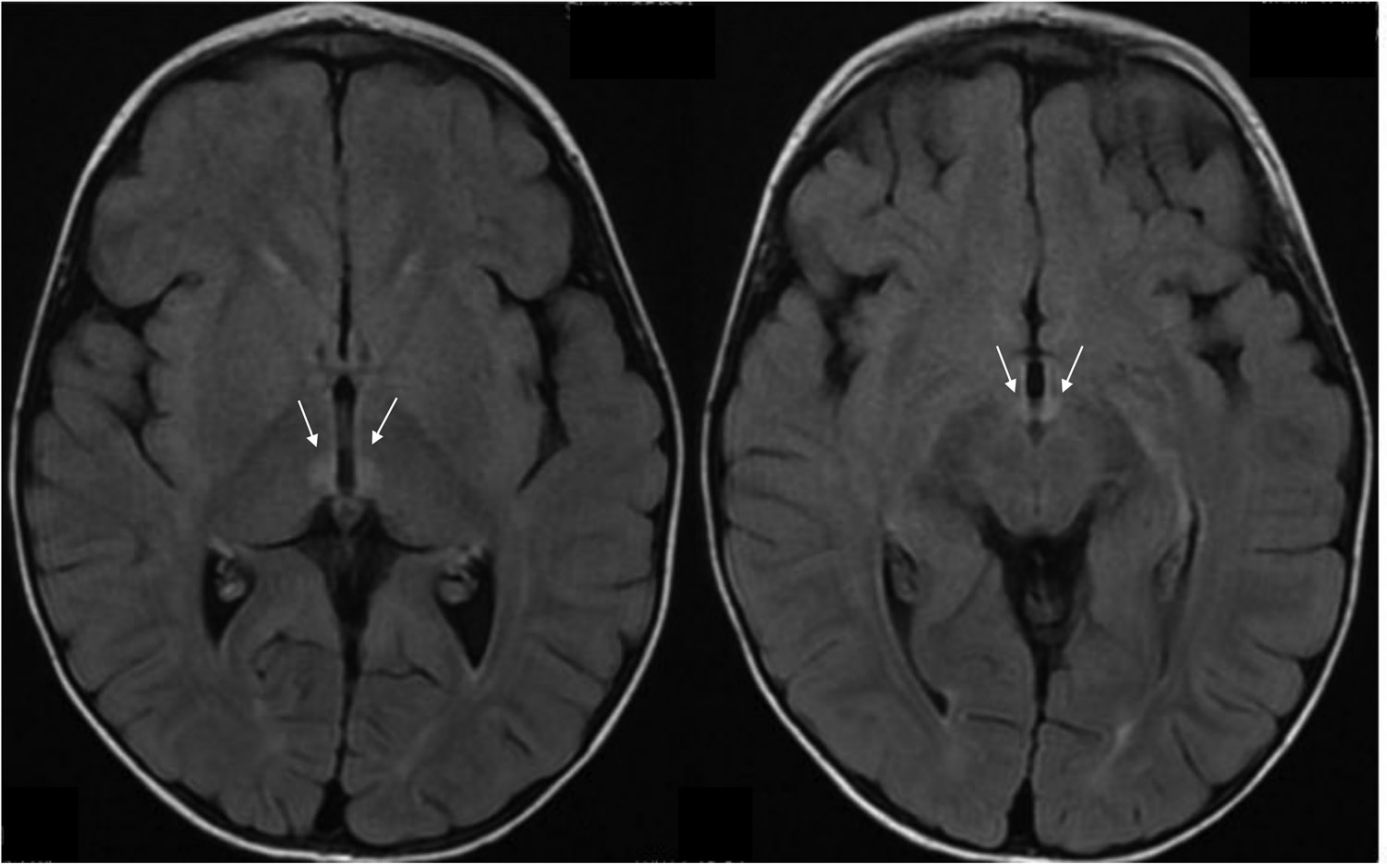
FLAIR sequence T2-weighted MRI of the brain with arrows indicating abnormal hyperintensity in the medial thalami (left) and mammillary bodies (right).
